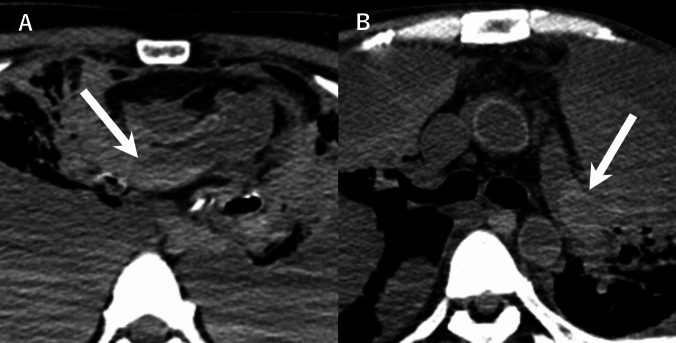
Examples of pulmonary arterial thrombosis diagnosed by autopsy. A 74-year-old female. Axial postmortem CT image showing dependent high-density area in the right pulmonary artery (arrow) that resembles gravitational sedimentation as a postmortem change. CT was performed 488 min after death. B 45-year-old male. Axial postmortem CT image showing high-density area along the vessel wall in the left pulmonary artery (arrow) that resembles cast-like blood clot as a postmortem change. CT was performed 128 min after death
